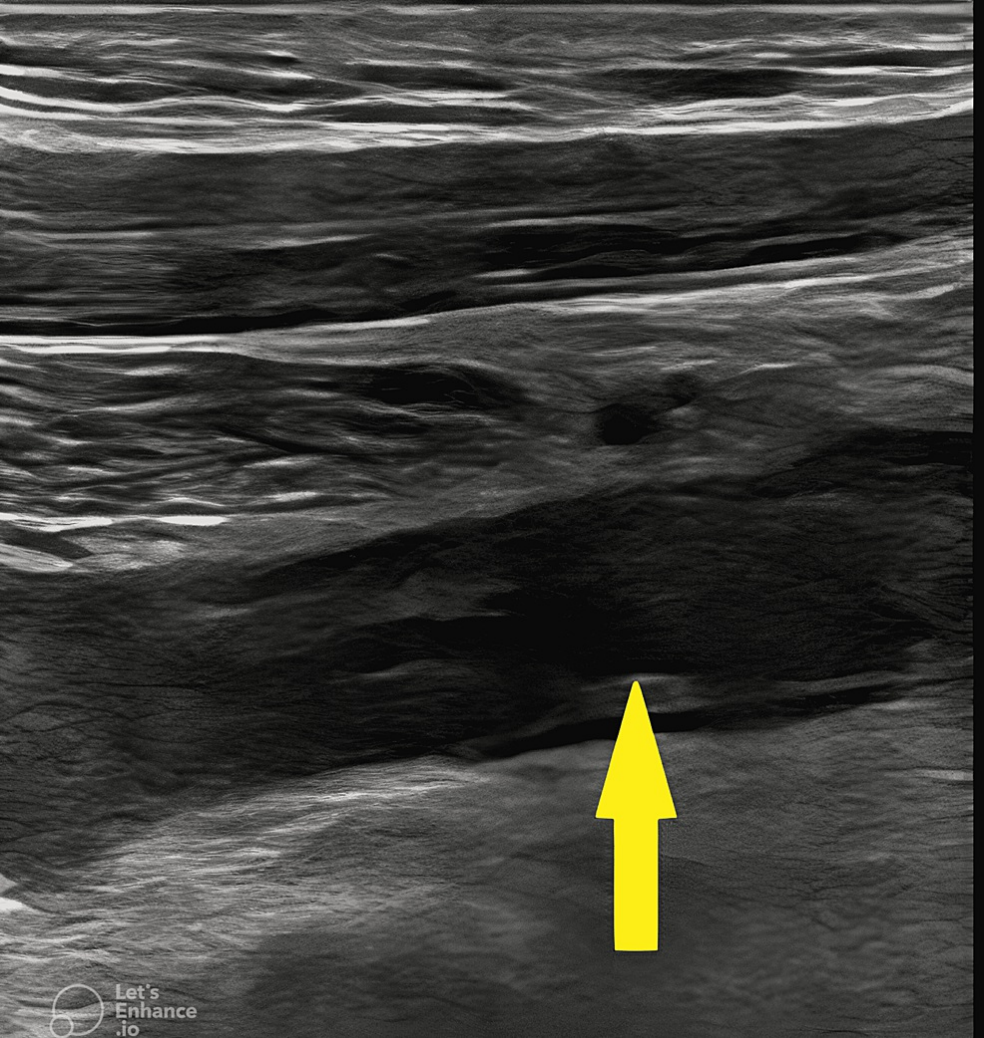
Ultrasound of right subclavian vein with thrombus (arrow).
